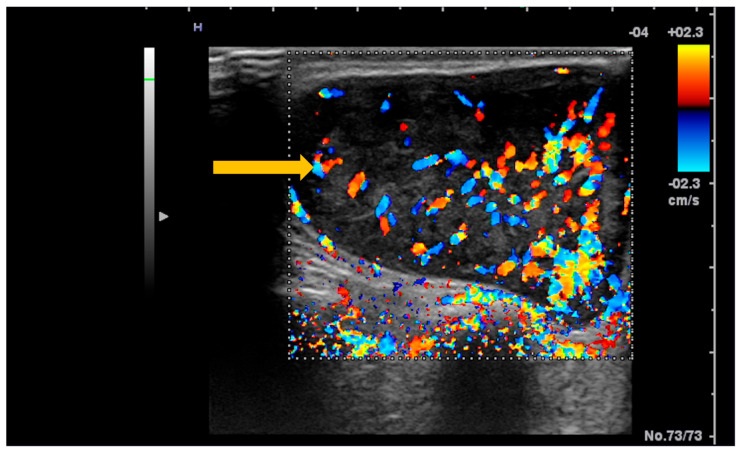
Color Doppler ultrasound of the parotid gland in a patient with Sjögren’s syndrome. Color Doppler imaging demonstrates a newly developed hypervascular nodular lesion within the parotid gland (arrow), a finding suspicious for salivary gland lymphoma in the clinical context of Sjögren’s syndrome.
